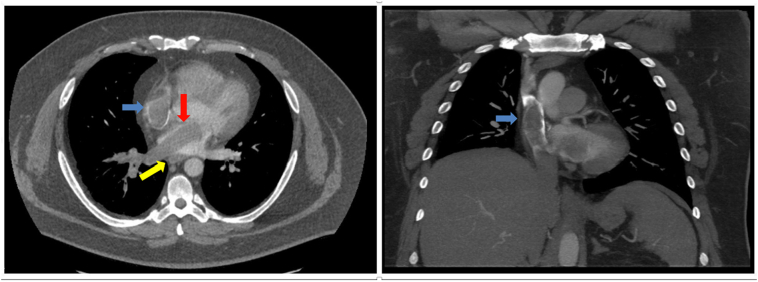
Axial and coronal view CTA revealing filling defect in the left atrium (yellow arrow), right pulmonary vein and artery (red arrow), and SVC (blue arrows).
